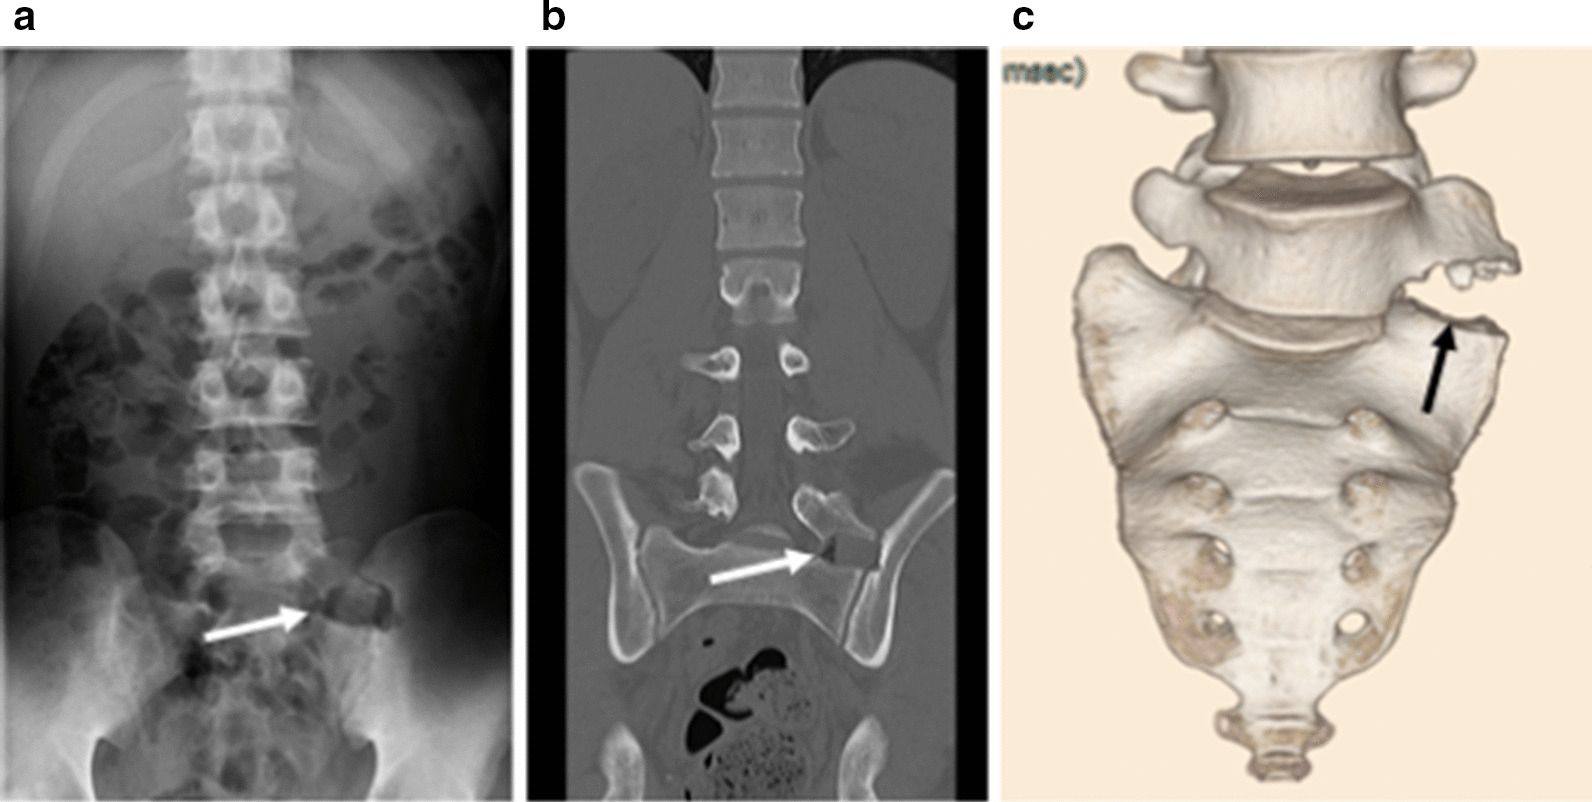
a Postoperative anteroposterior plain radiography. b Postoperative coronal computed tomography (CT) image. c Postoperative three-dimensional (3D) CT image. Arrow indicates the removal of pseudoarticulation
